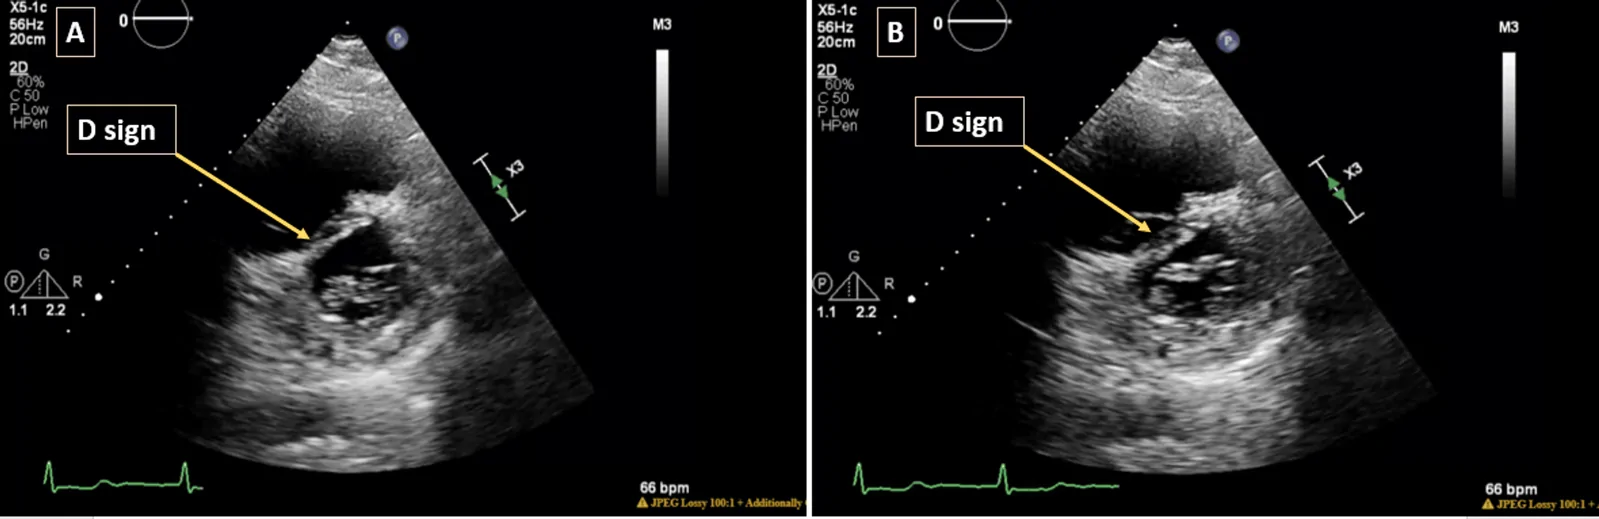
Parasternal short-axis views demonstrating persistent interventricular septal flattening consistent with right ventricular pressure overload (A) End-systolic parasternal short-axis view demonstrating a D-shaped left ventricle due to interventricular septal flattening; (B) End-diastolic parasternal short-axis view demonstrating persistent interventricular septal flattening with a D-shaped left ventricle
